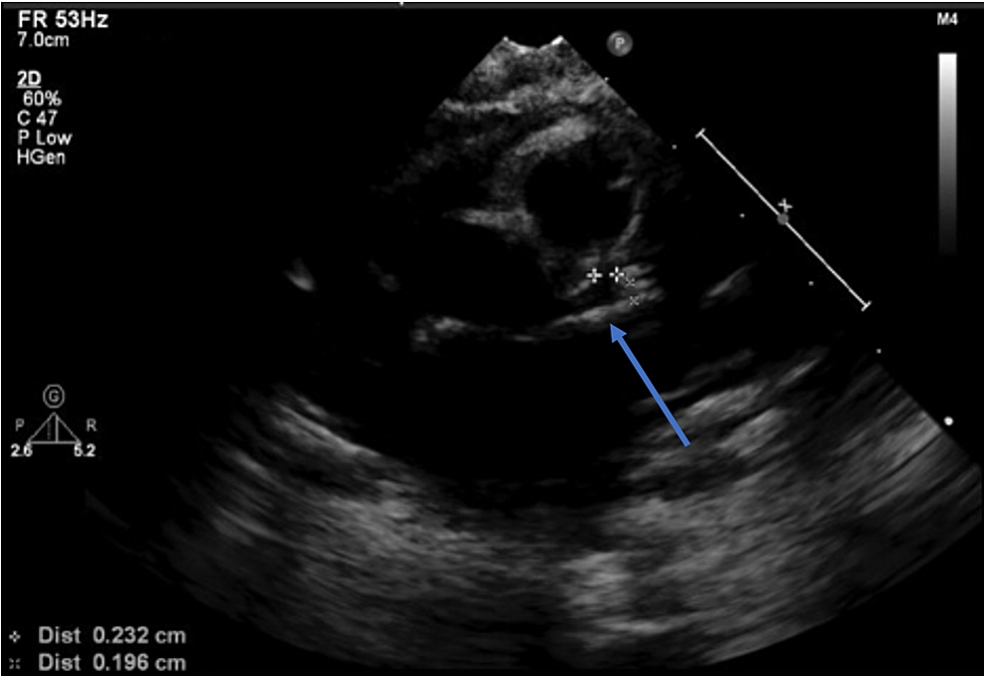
Transthoracic echocardiogram obtained at three months of illness showing resolution of left main coronary artery dilatation (blue arrow); left coronary artery ostium marked with plus markers.
